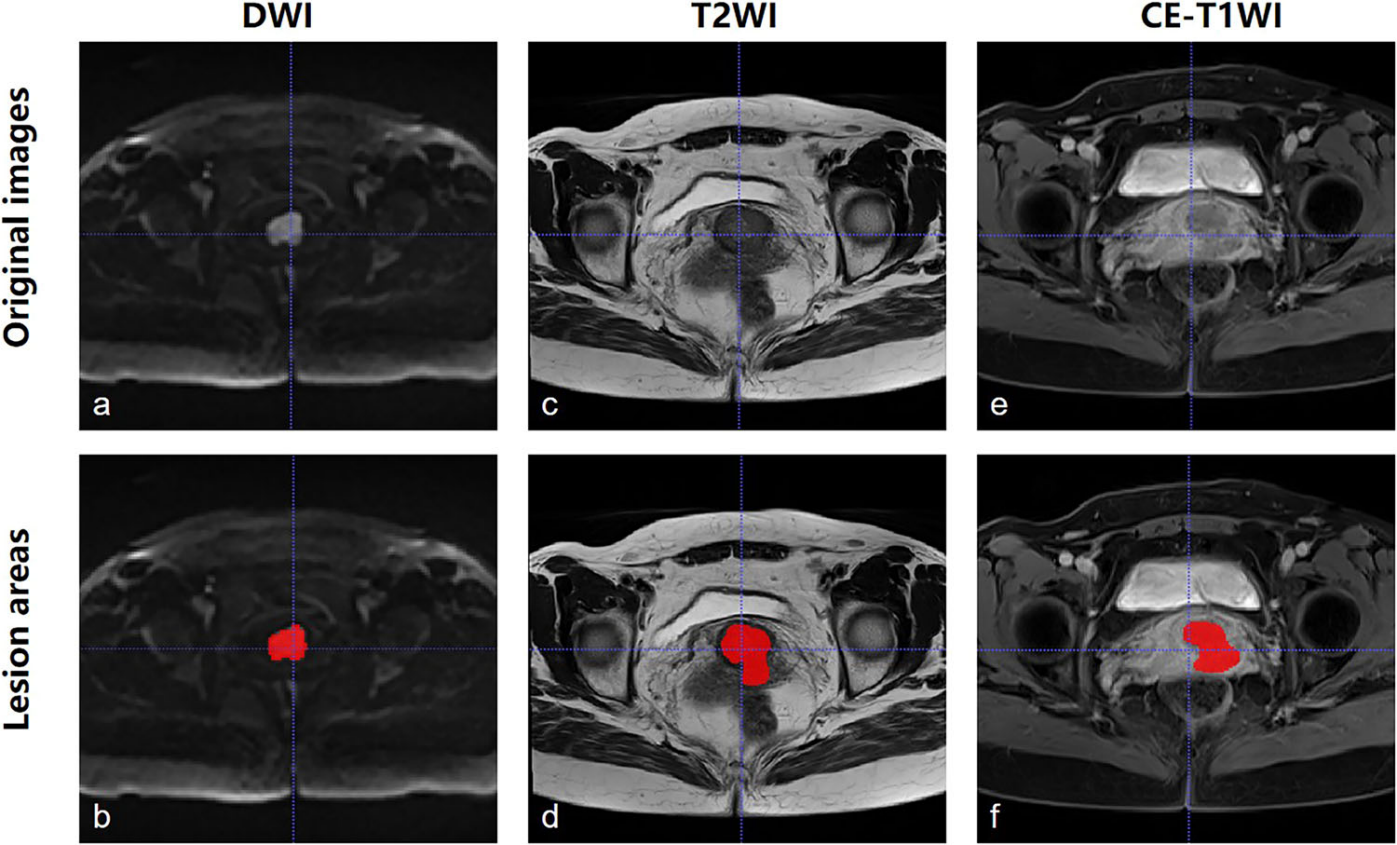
Example images from a 49-year-old woman with cervical cancer on DWI, T2WI, and CE-T1WI, with corresponding radiologist-delineated lesion regions. a, b DWI image and ROI image, *b* = 1,000 s/mm^2^. c, d T2WI image and ROI image. e, f CE-T1WI image and ROI image. CE-T1WI, Contrast-enhanced T1-weighted imaging; DWI, Diffusion-weighted imaging; ROI, Region of interest; T2WI, T2-weighted imaging
